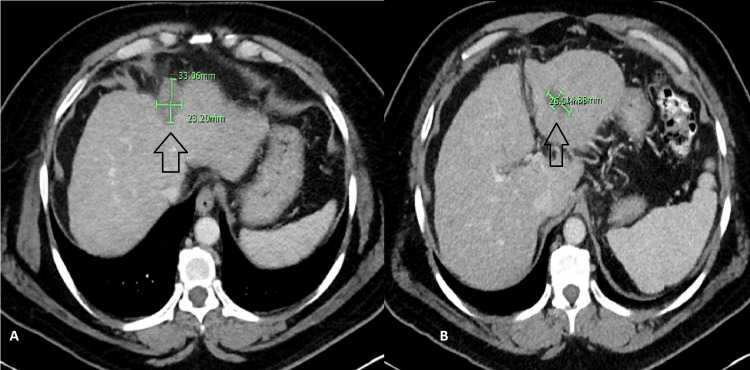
February 2021 CT scan of the abdomen Further evidence of the radiological response of the masses is observed (arrows in A and B)
